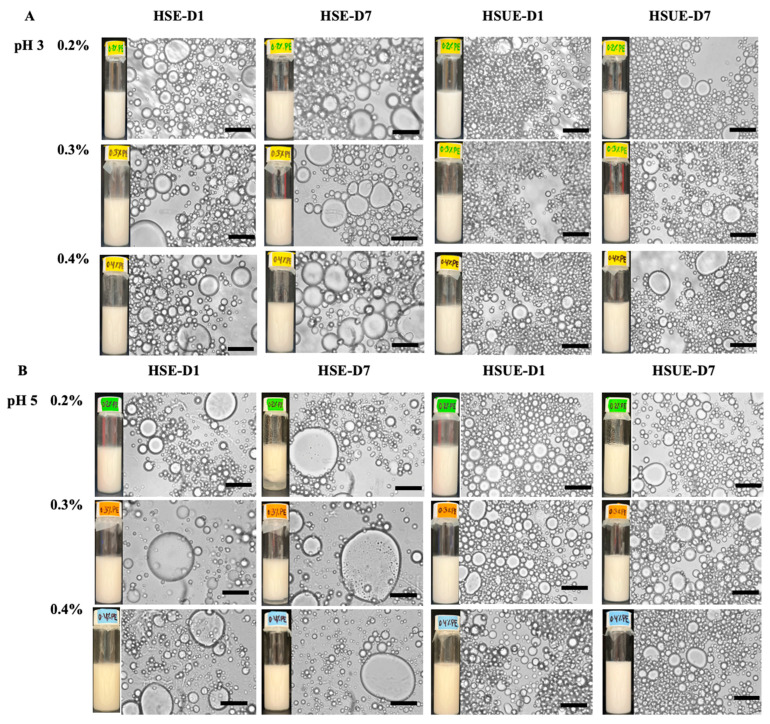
Microstructure and visual observation images of T-CNC-based Pickering emulsions at (A) pH 3 and (B) pH 5 stabilized with different concentrations of T-CNC (0.2–0.4%, *w*/*w*) on day 1 (D1) and day 7 (D7). Emulsions were prepared by high-shear homogenization (HSE) and by high-shear homogenization followed by ultrasonication (HSUE). Scale bars represent 10 μm.
